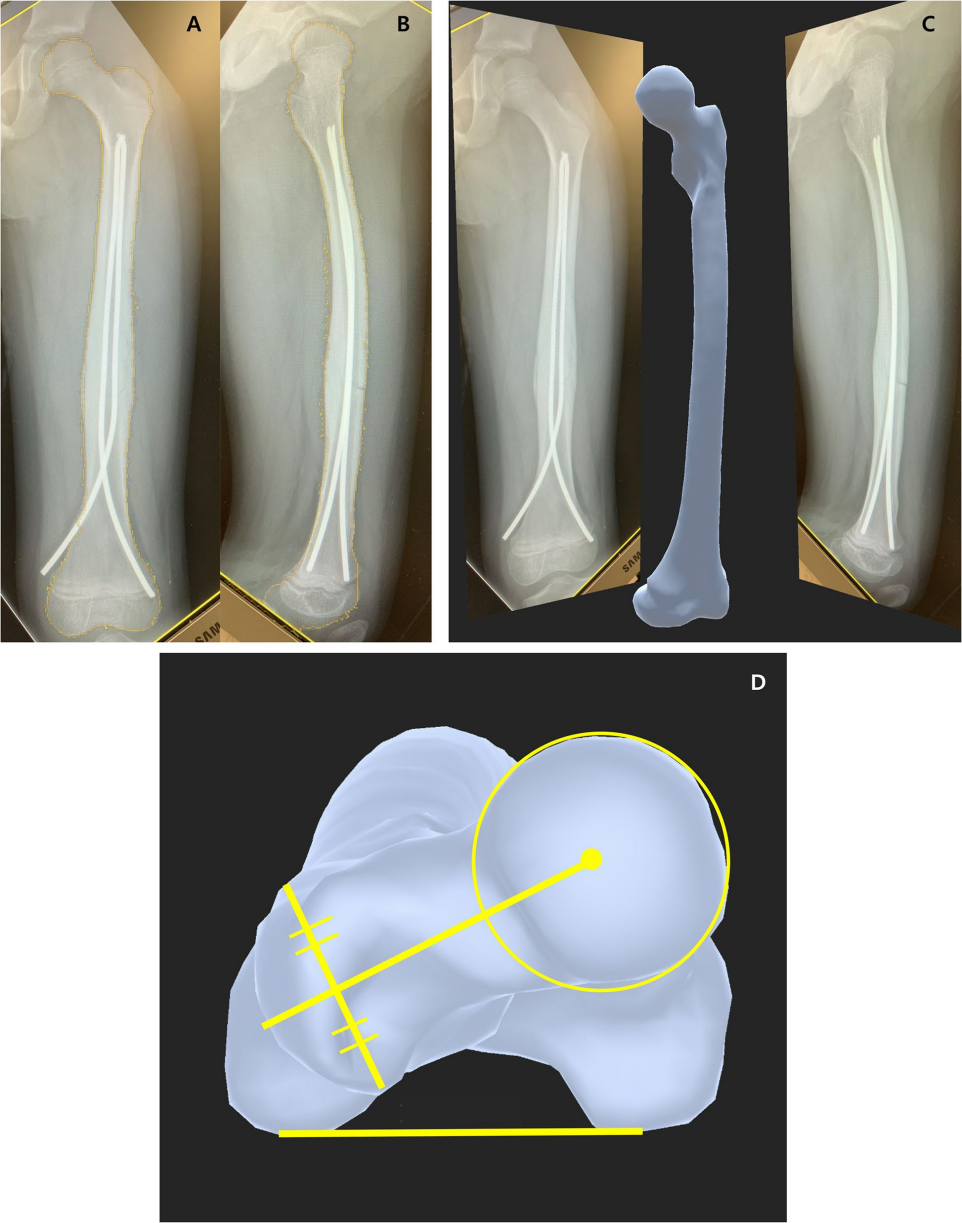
Three-dimensional reconstruction using the statistical shape model (Femora®). This is performed by outlining the bony cortices, allowing accurate measurement even when metal implants are present (A, B). A three-dimensional reconstruct is obtained from two-dimensional uncalibrated plain radiographs (C). Femoral anteversion can be measured by measuring the angle between a line connecting the femoral head center and neck, and another line connecting the posterior femoral condyles (D)
